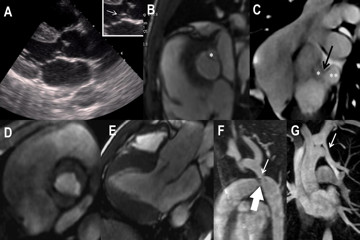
Imaging findings of subvalvular aneurysm and associated congenital defects. (A) Echocardiogram in the plane of left ventricular outflow tract (LVOT) observed (highlighted) an aneurysm formation in the topography of subvalvular mitral–aortic fibrosa (arrow). (B, C, D and E) Cine-cardiovascular magnetic resonance to study the region of mitral–aortic intervalvular fibrosa. (B) Subaortic plane showing inner portion (*) of subvalvular pseudoaneurysm. (C) Coronal oblique plane, specific to the topography of the lesion, where the inner portion (*), neck (black arrow) and intrapericardial portion () of the pseudoaneurysm can be observed. (D) Valve plane during systole showing the opened bicuspid aortic valve. (E) LVOT plane individualizing mild aortic regurgitation, this plane does not have the same angle of echocardiography and therefore does not demonstrate the lesion. (F and G) Magnetic resonance angiography of the thorax. (F) Patent arteriosus ductus (large arrow) and coarctation of the aorta (arrow). (G) Anomalous pulmonary venous return of the left upper lobe vein into the innominate artery on the same side (arrow).
